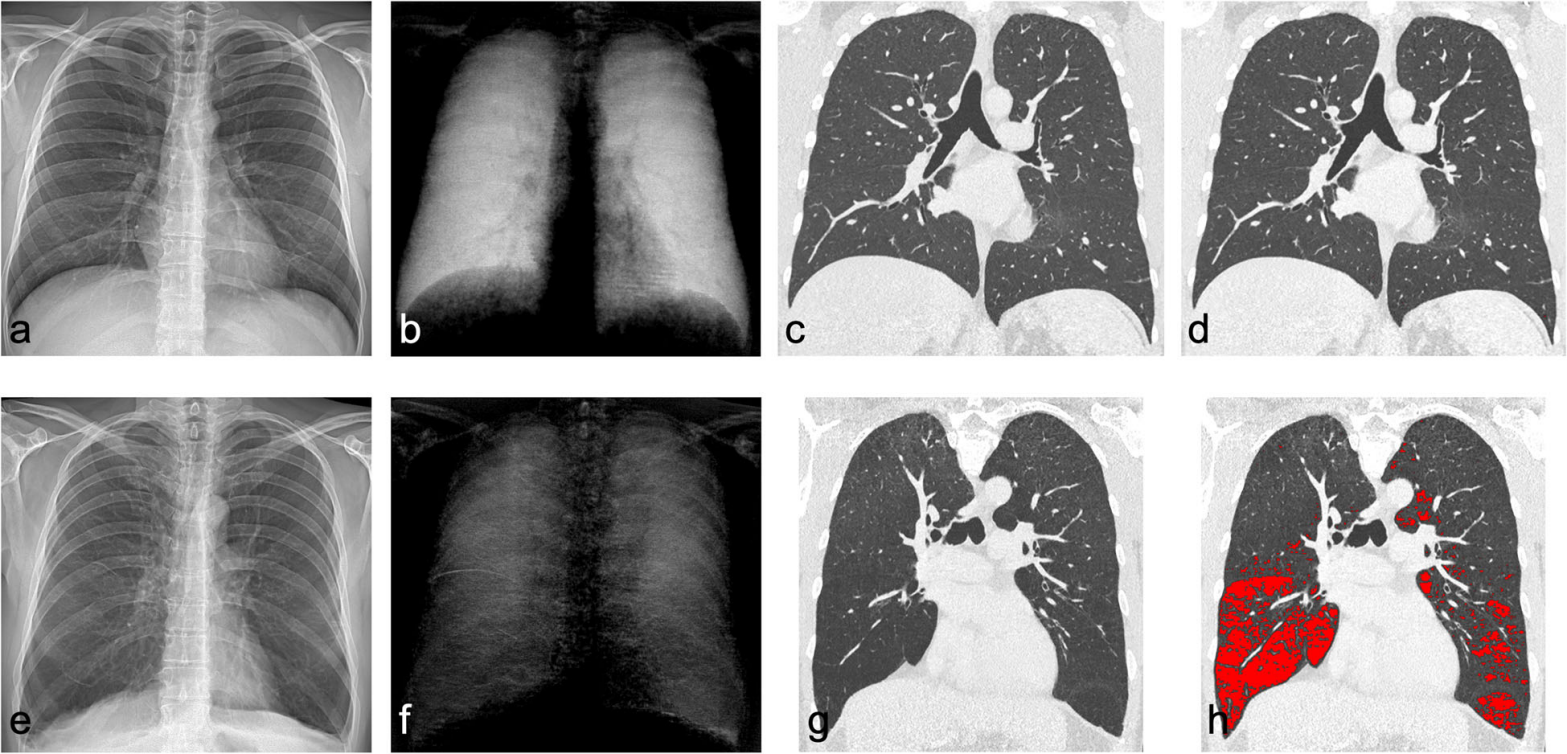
Conventional (a) and dark-field chest x-ray (b) of a healthy subject and a patient (#5) with alpha1-antitrypsin deficiency (AATD) (e, f). For both subjects, same window/level settings were applied within conventional and dark-field images, respectively. The dark-field signal is notably reduced in the lungs of the AATD patient (f), in particular in lower zones, whereas healthy lungs show a strong homogenous signal over all zones. Corresponding coronal computed tomography (CT) image (g) reveals lung parenchymal destruction, highlighted by emphysema overlay (red colour) using a threshold of -950 Hounsfield units (h) compared to images of healthy patient (c, d). Conventional chest x-ray fails to visualise extent of parenchymal disease in the AATD patient (e), showing similar transmission in the healthy patient (a). Only secondary signs like flattening of diaphragm, increased volume of lungs or widened costophrenic angles point to emphysema (e). While the dark-field image exhibits a generally reduced dark-field signal over the entire lung region in contrast to the normal lung, CT only reveals defects in lower regions which correspond to the stronger manifestation of signal reduction in the dark-field radiograph
